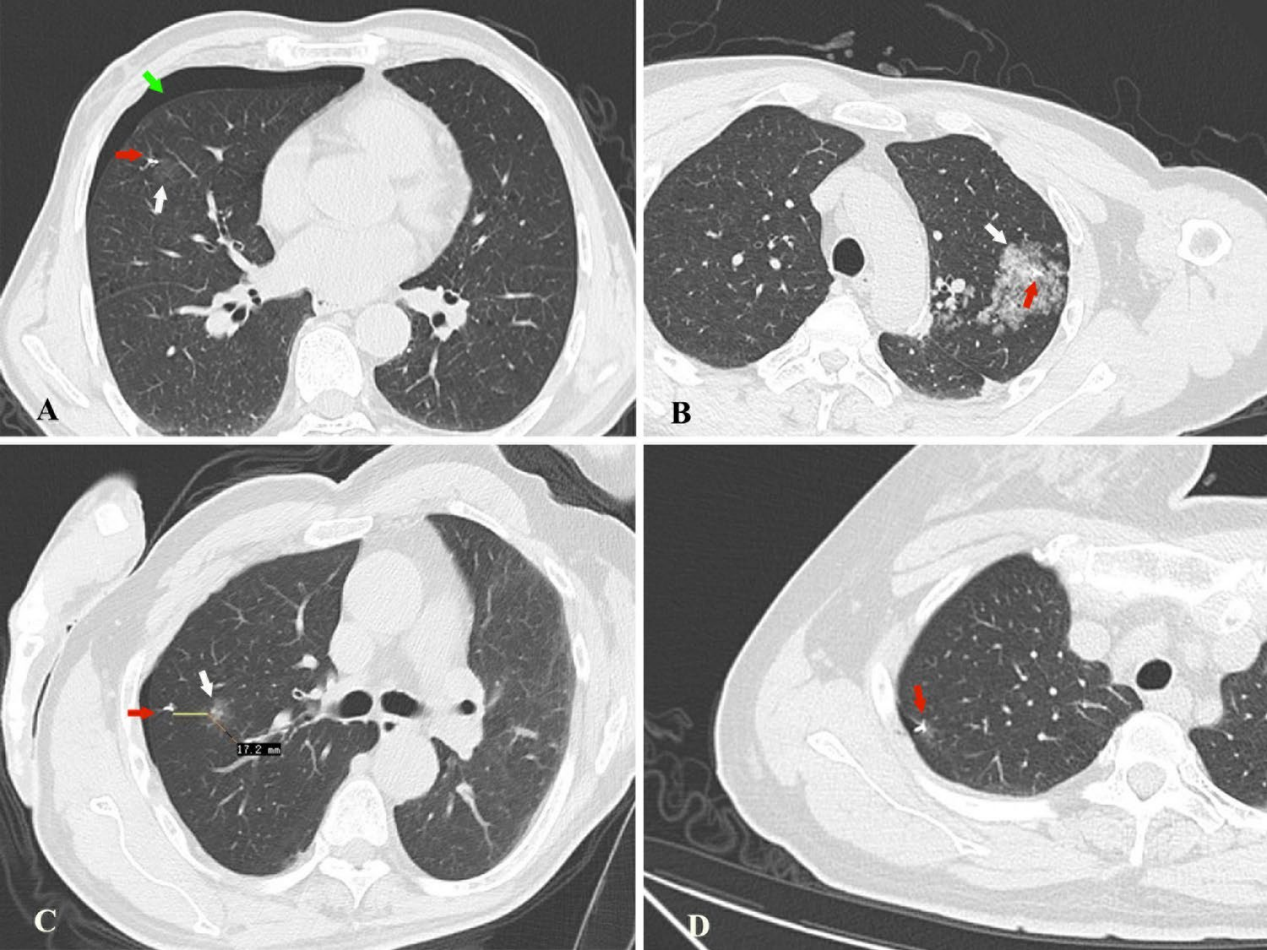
Complications and unsuccessful localization. (A) CT image shows asymptomatic pneumothorax (green arrow) with the claw (red arrow) and nodule (white arrow) in right middle lobe. (B) CT image shows asymptomatic parenchymal hemorrhage (white arrow) with the claw (red arrow) in left upper lobe. (C) CT image shows the distance between the nodule and claw was more than 1 cm with the claw (red arrow) and nodule (white arrow) in right upper lobe. (D) CT image shows displaced claw (red arrow) pushing against the lung tissue in right upper lobe.
